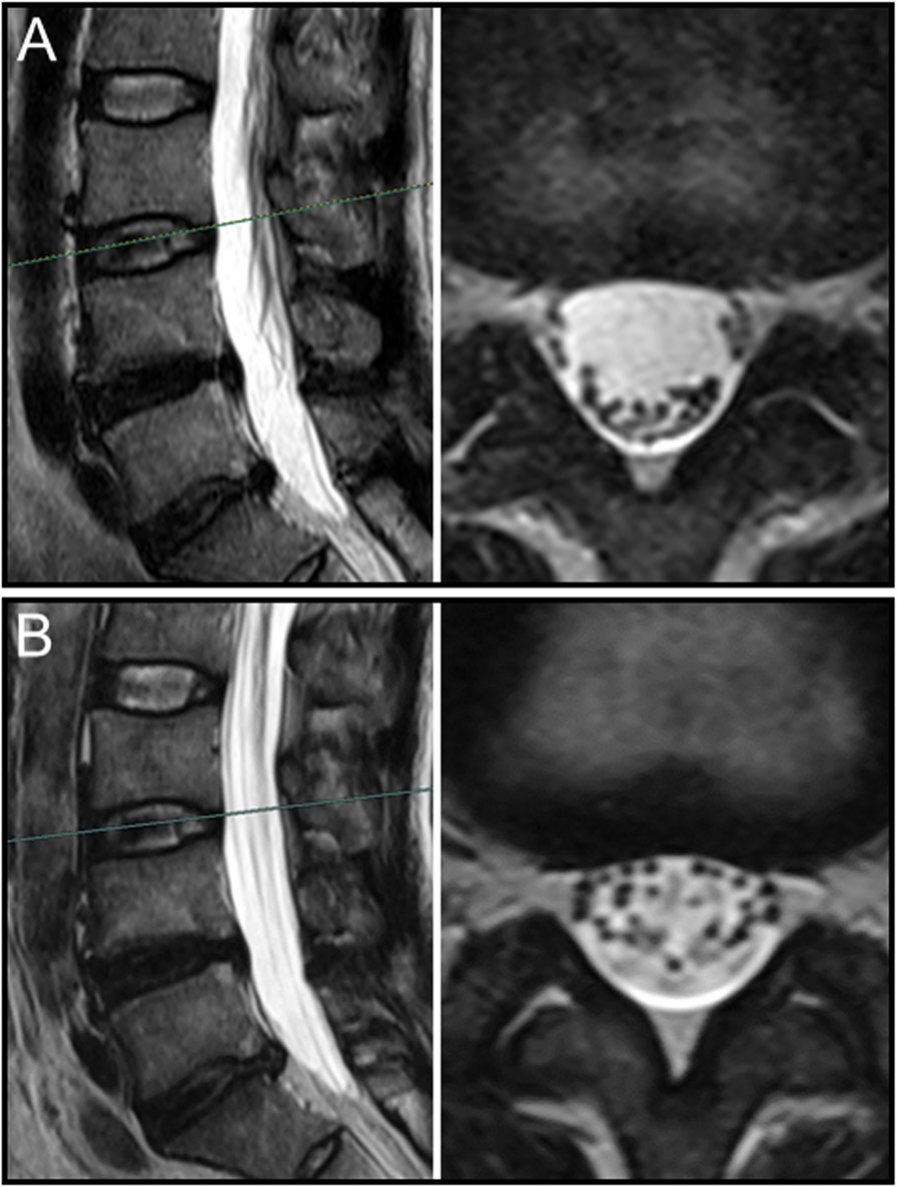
In a man with negative SedSign (a), the nerve roots did not subside to the ventral side of dural sac but floating in the middle of dural sac on prone MR images (b)
